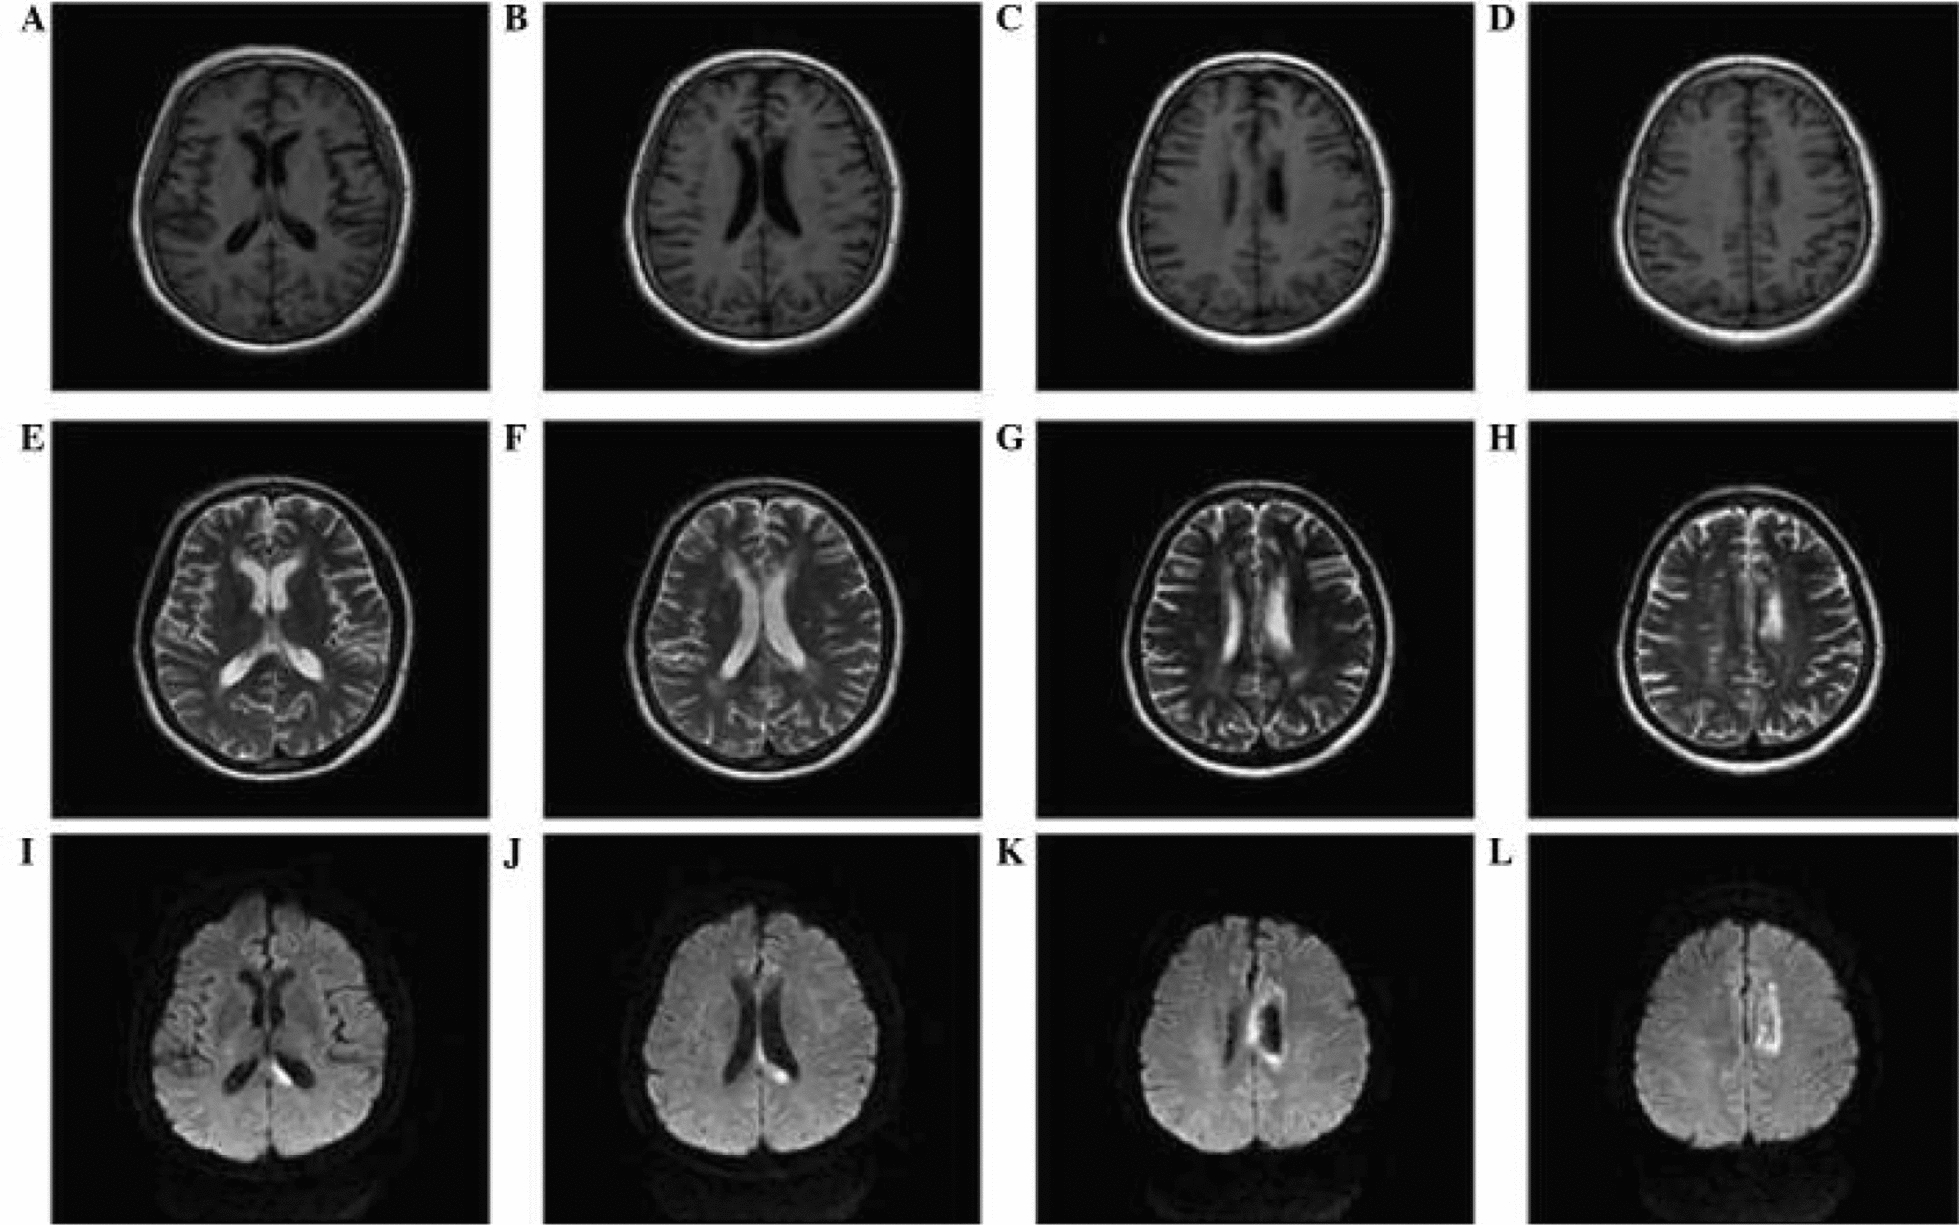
Multimodal MRI of the left corpus callosum: T1 (hypo-intensity in the splenium  (A–B) & body (C–D)), T2 (hyper-intensity in the splenium  (E–F) & body (G–H)), and diffusion-weighted (high signal intensity in the splenium (I–J) & body (K–L)) images confirming infarction [15]
